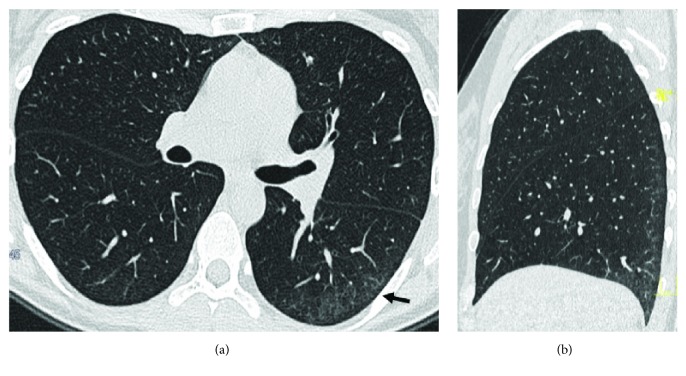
A 36-year-old woman with UC and previous total colectomy. In the basal posterolateral segment of lower lobes (more evident in the left), a fine interstitial thickening associated with ground glass opacities and some traction bronchiolectasis is appreciable. A subpleural sparing is associated (arrow). These findings are consistent with NSIP. Axial HRCT study (a) and sagittal reconstruction (b).
